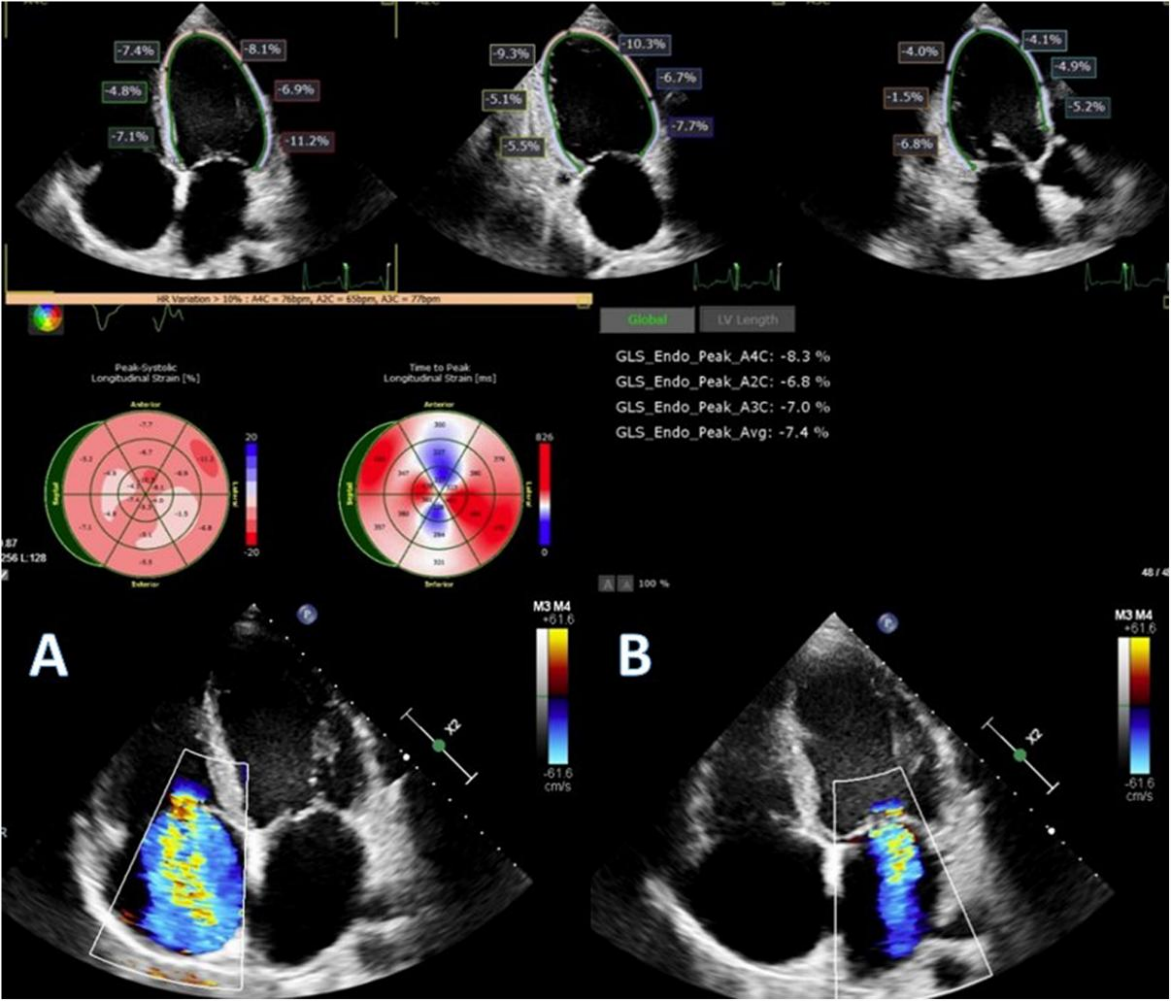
2D echocardiography: left ventricular longitudinal strain measurement (apical position four-chamber, two-chamber, and three-chamber views; strain values in the middle ‘bull’s-eye’ view). (*A*) Four-chamber view of the apical position: dilatation of all cardiac chambers, secondary large tricuspid regurgitation by colour Doppler; (*B*) apical position four-chamber view: colour Doppler—secondary moderate mitral regurgitation.
